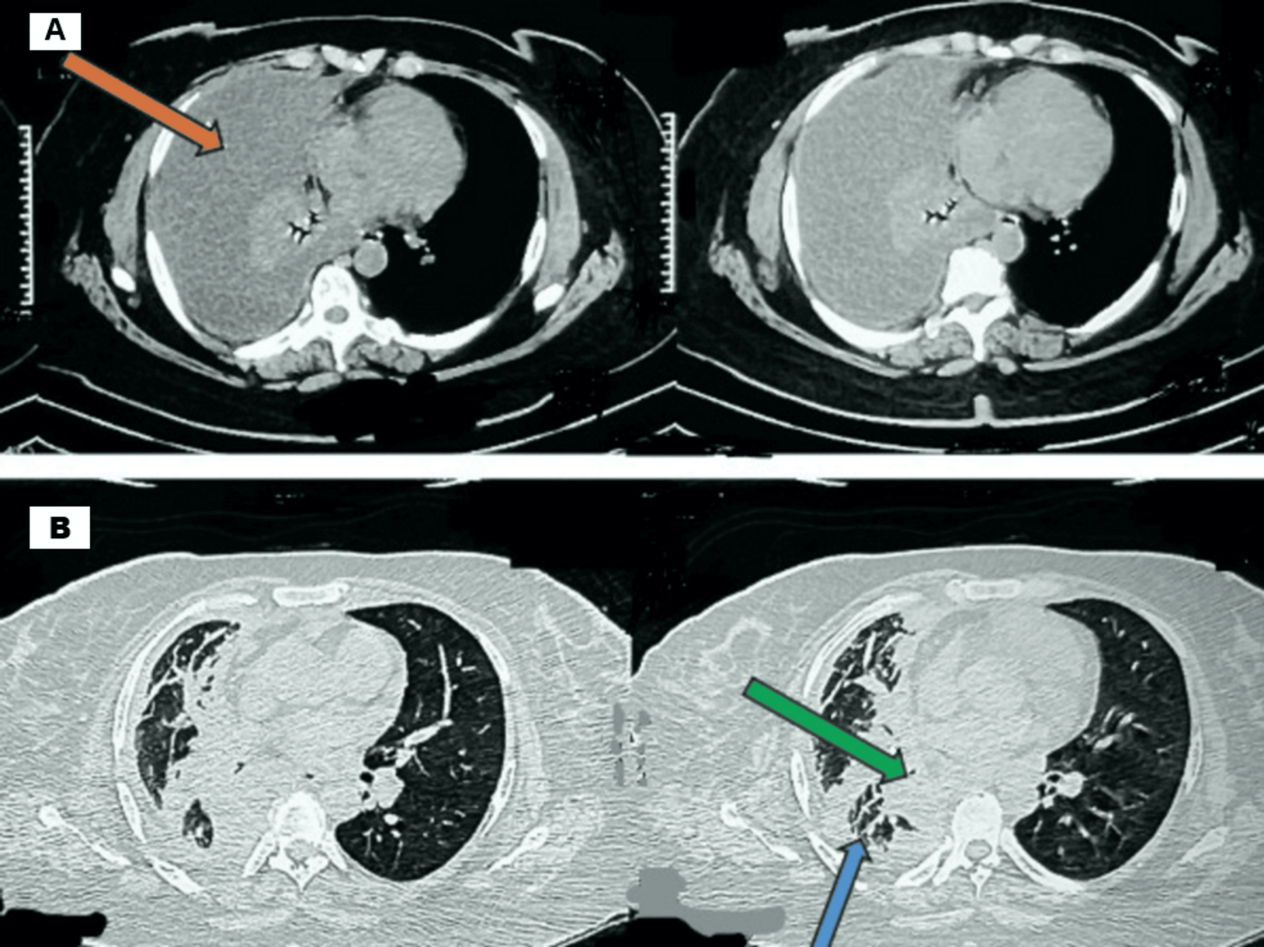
Baseline CT chest of the patient CT chest showing right-sided moderate pleural effusion with pleural thickening (orange arrow), right parahilar lesion (green arrow), and right lower lobe nodular septal thickening (blue arrow) (A) Mediastinal window image; (B) parenchymal window image CT: computed tomography
